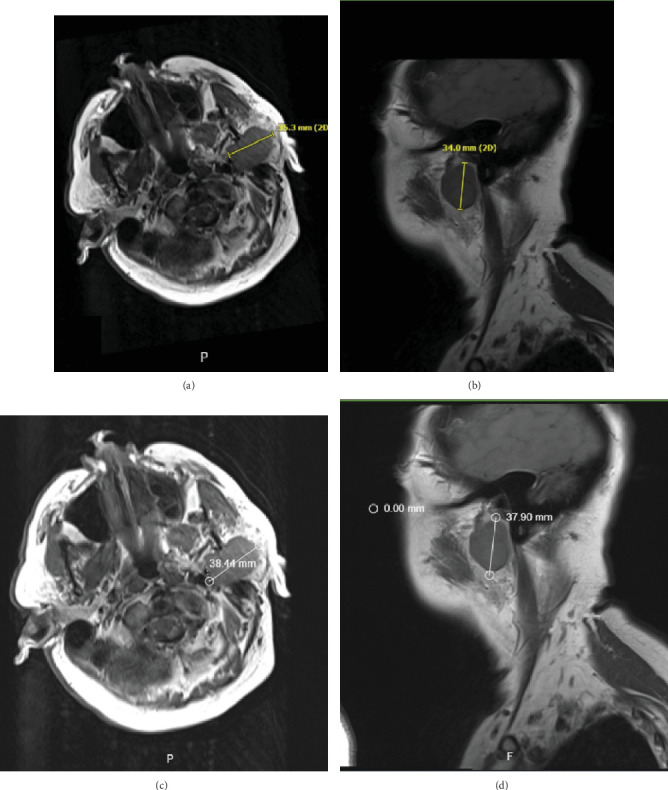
MRI soft tissue neck axial and sagittal T1 weighted images. (a, b) Left parotid mass at the time of initial workup of left jaw pain. (c, d) Rapid growth of parotid mass 10 days after initial diagnosis.
